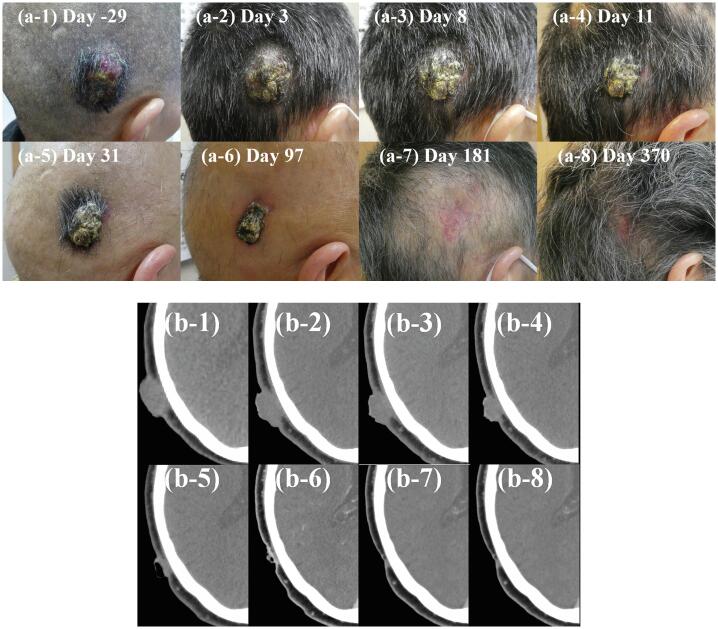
Time course of Patient 1 tumor before and after boron neutron capture therapy (BNCT). (a) Clinical photos and (b) Computed tomography scans obtained before treatment start (day −29) and on treatment days 3, 8, 11, 31, 97, 181, and 370. The patient achieved PR and CR on days 31 and 181, respectively, according to the RECIST 1.1 criteria.
